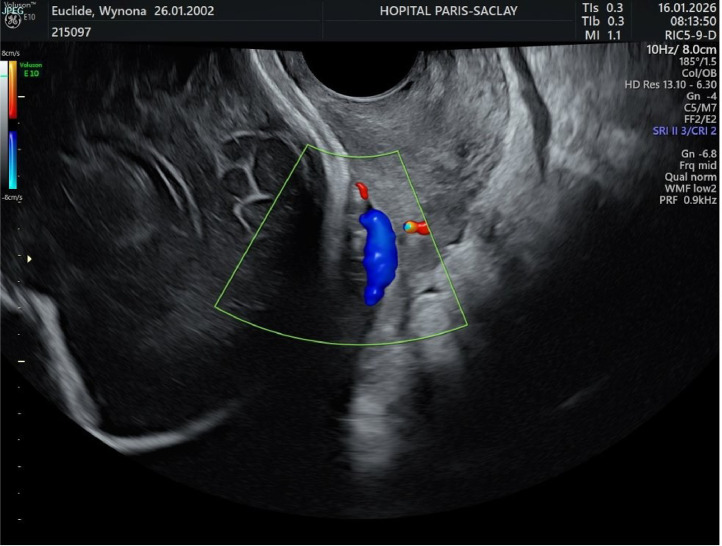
Transvaginal ultrasound with color Doppler imaging at 34 weeks and 6 days confirming vasa previa. The image shows fetal vessels with characteristic arterial (red) and venous (blue) flow patterns crossing directly over the internal cervical os. This color Doppler appearance provides definitive confirmation of vasa previa with the vessels at high risk of rupture during labor or membrane rupture.
